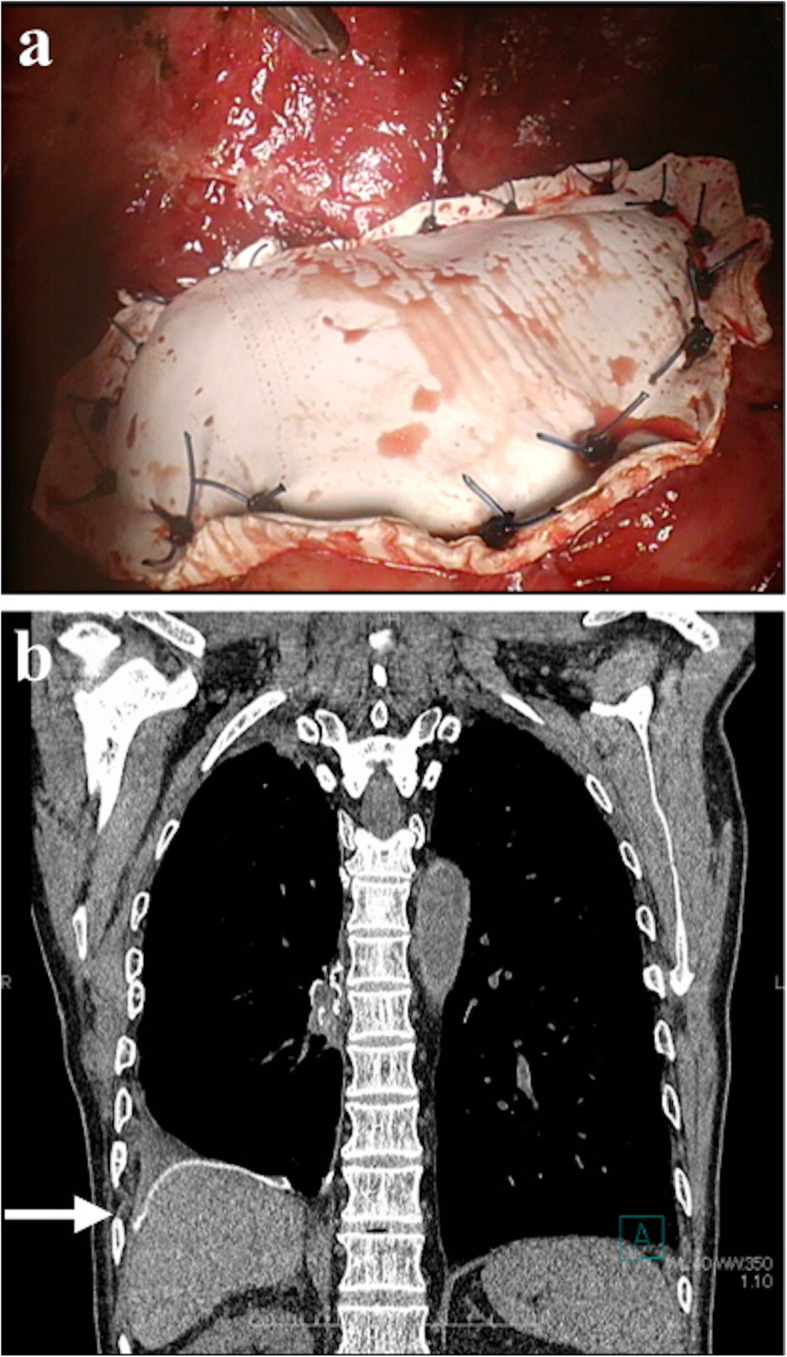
Case 1. A 76-year-old man with primary lung cancer of the right lower lobe invading the right diaphragm and liver. (a) Intraoperative view. Part of the diaphragm was reconstructed with GORE DUALMESH. (b) Computed tomography 1 year after surgery. The mesh remains in place without any sign of complication (white arrow)
